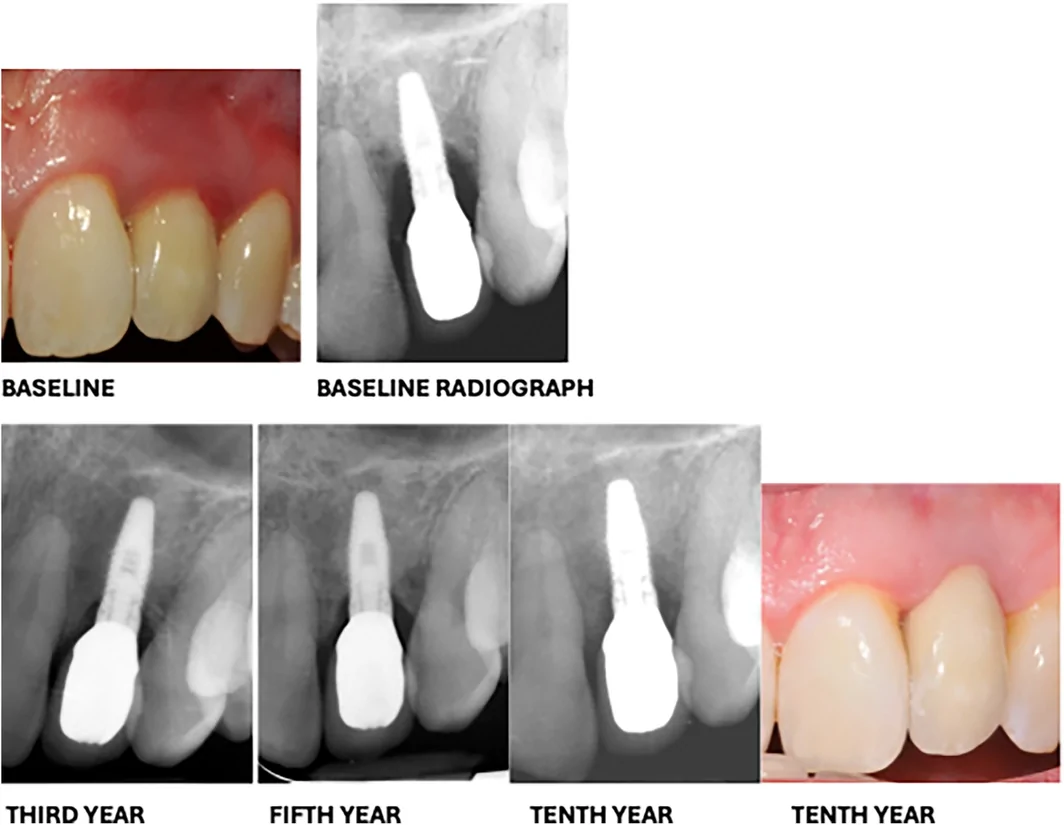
Radiographs acquired at the baseline assessment, as well as those recorded during the evaluations conducted in the 3rd, 5th and 10th years.
